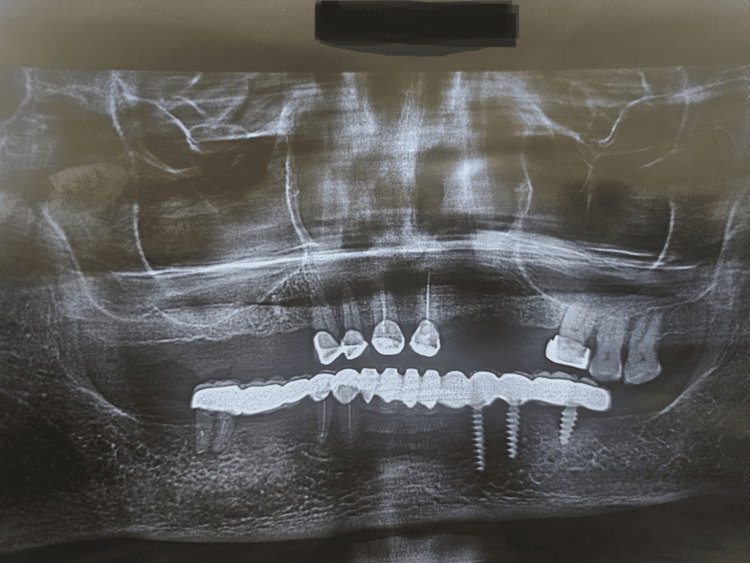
Post-insertion orthopantomogram (OPG) of the patient.
